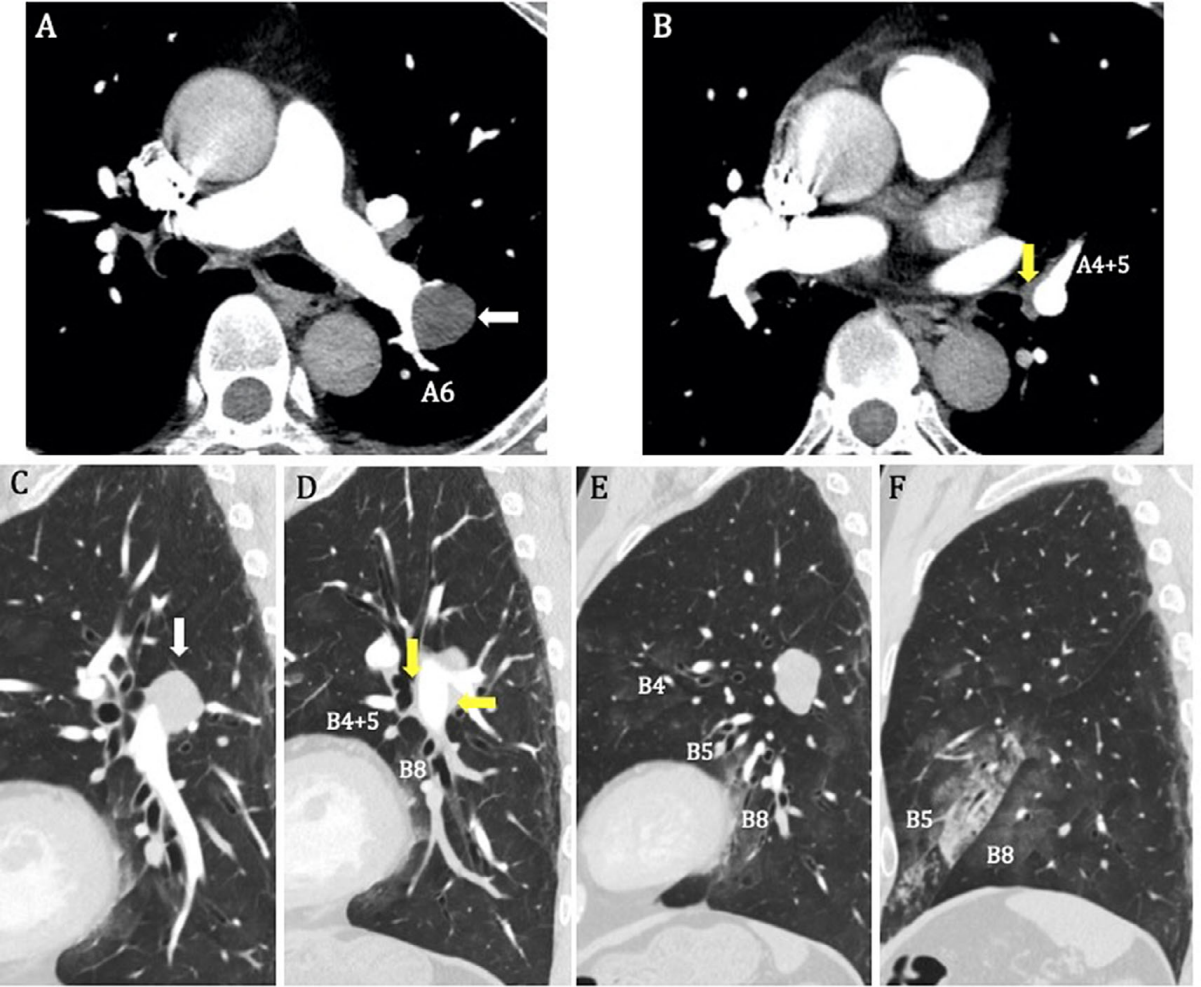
(A–D) Enhanced chest computed tomography (CT) shows a tumour measuring 25 × 24 mm in the left lower lobe (white arrow) with fluid accumulation, which suggests bleeding around the main trunk of the pulmonary artery (yellow arrow). (E, F) Enhanced chest CT showing a consolidation mainly in the lingular segment of the left lung.
